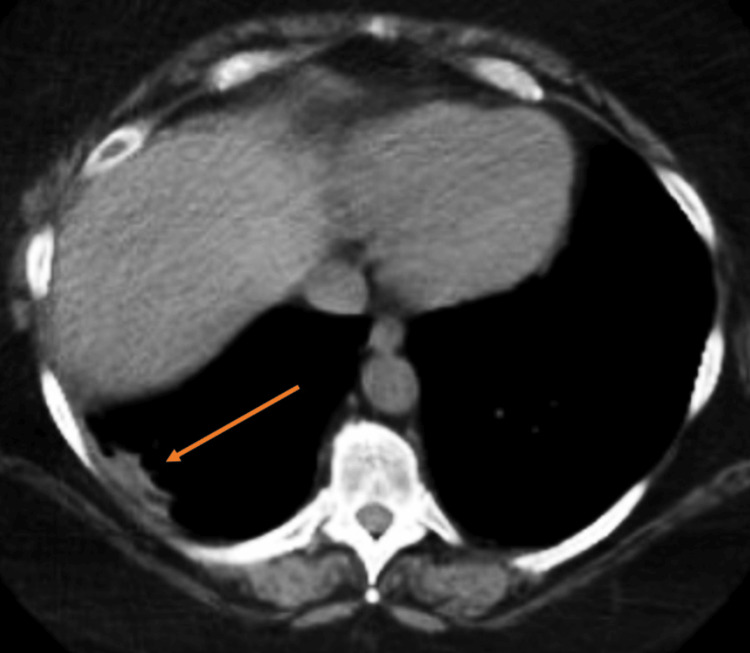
Follow-up CT Marked improvement from previously noted thick-walled cavity.
